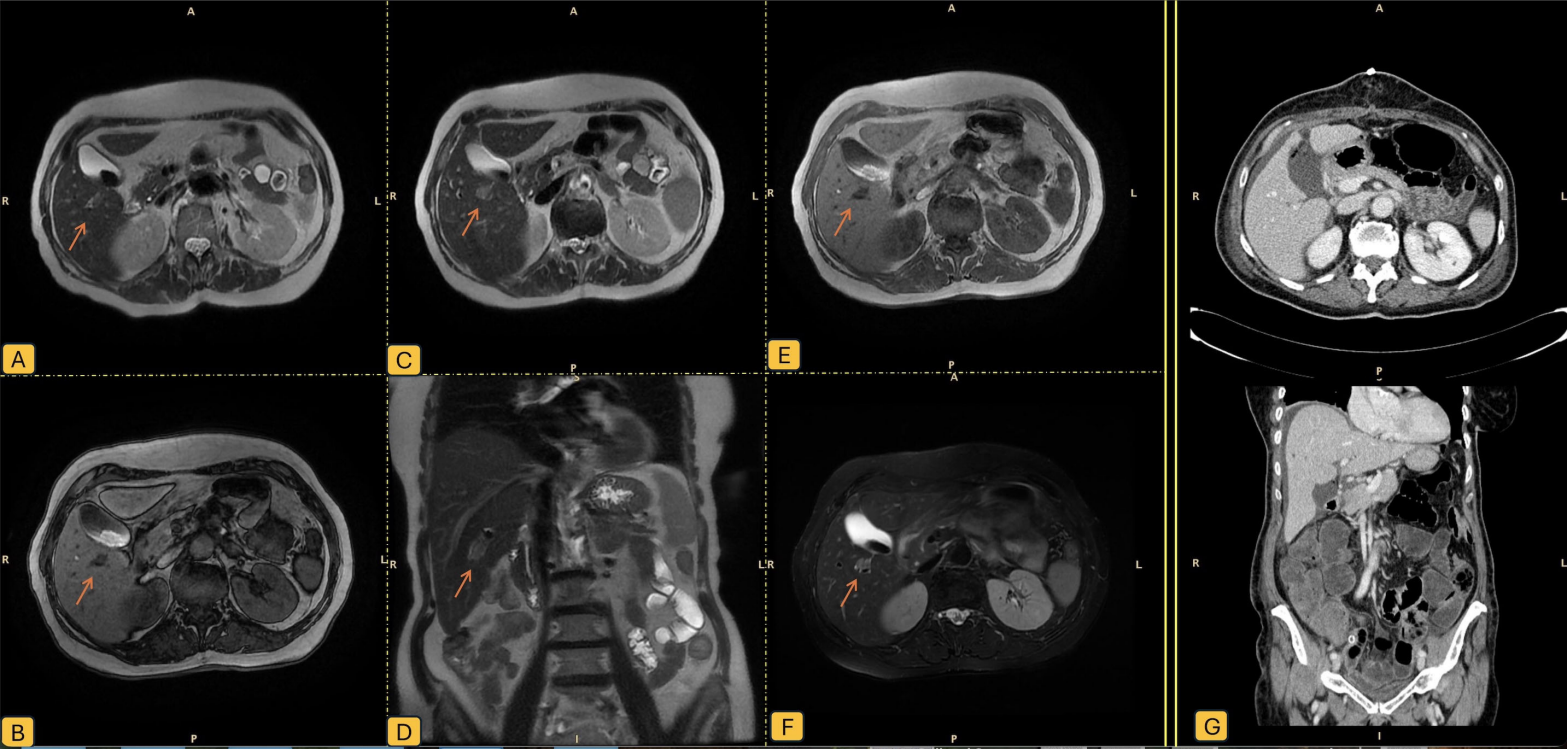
Liver lesion at diagnostic imaging; (A) T2-weighted images using single shot fast spin echo sequence (SSFE); (B) T1-weighted sequence; (C) another T2 SSFSE sequence ; (D) coronal T2 SSFE; (E) T1-weighted gradient-echo in-phase; (F) T2 Fast Spin Echo with Fat Saturation (FRFSE FATSAT); (G) TC scan after cytoreductive surgery. Orange arrow shows the epatic nodule.
